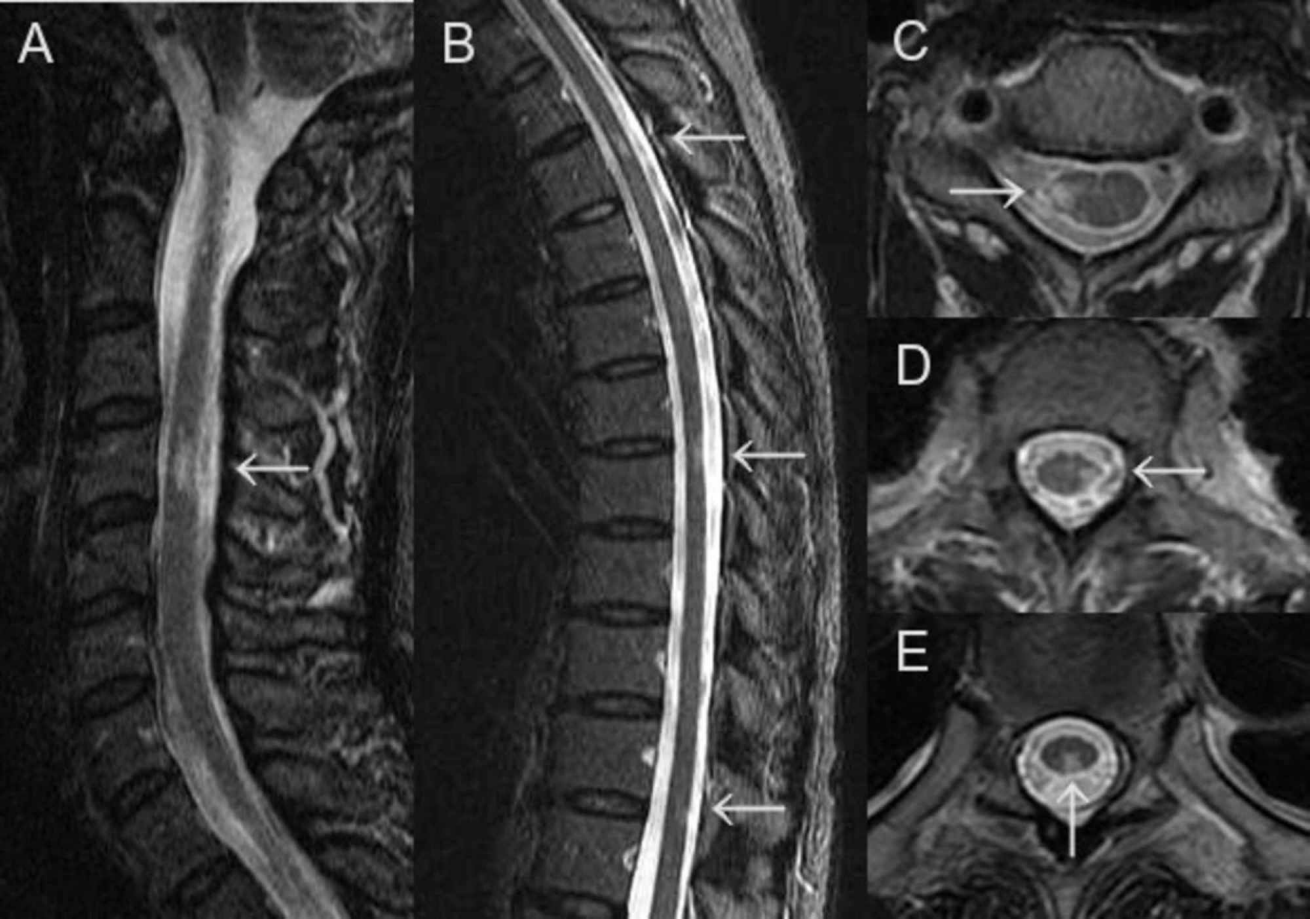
Spinal cord MRI findings. In spinal cord MRI, old short lesions of the cervical (A) and thoracic (B) spinal cord were observed at the central part in C3/4, the right side in C4 (C), the left lateral funiculus in T1/2 (D), the central part and posterior funiculus from T4 to T5, the posterior funiculus in T7/8 (E) and the posterior funiculus in T11/12.
